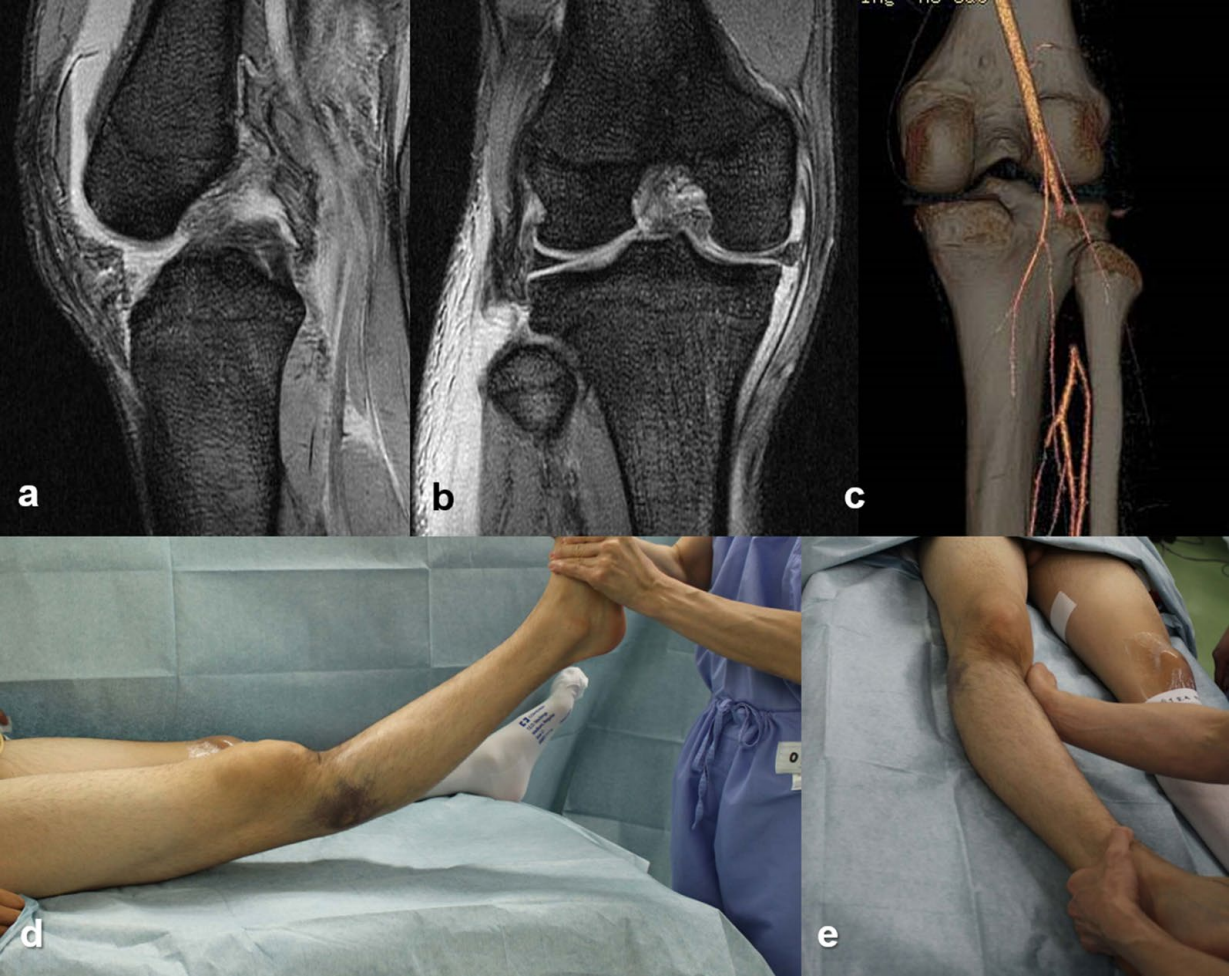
MRI and CT angiography and evaluation under anesthesia. An 18-year-old baseball player who suffered multiligament knee injuries during a baseball game. a Sagittal MRI showing PCL injury and posterior capsular injury; b coronal MRI revealing avulsion of the posterolateral complex from the fibular head; c angio-CT revealing occlusion of the popliteal artery; d, e evaluation under anesthesia showing severe posterior and varus instability
